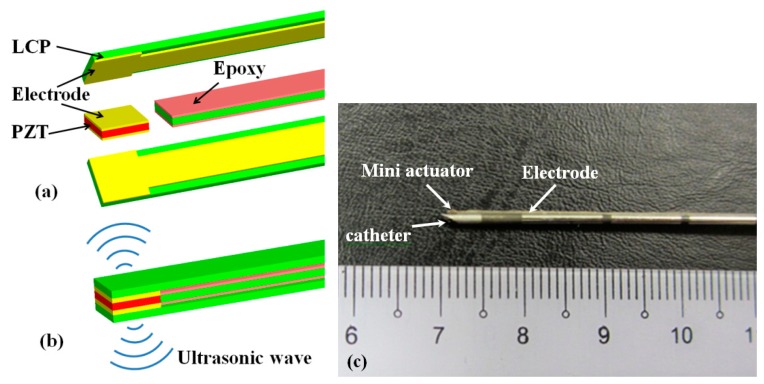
Sketches of: (a) the lead zirconate titanate (PZT) element being bonded between two flexible electrodes patterned on LCPs and (b) the assembled device; and (c) a photo of the ultrasound mini actuator attached to an 18 G biopsy needle.
